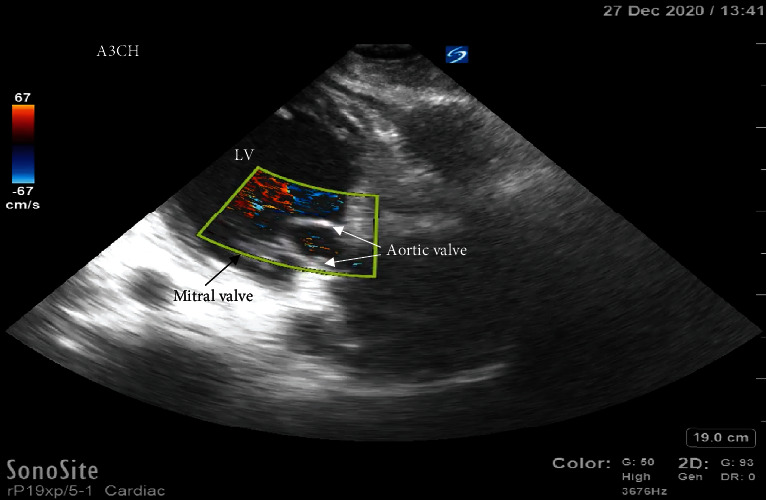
Color flow Doppler demonstrating regurgitant flow through the aortic valve, which has retracted back into the left ventricle because of the aortic root dissection.
